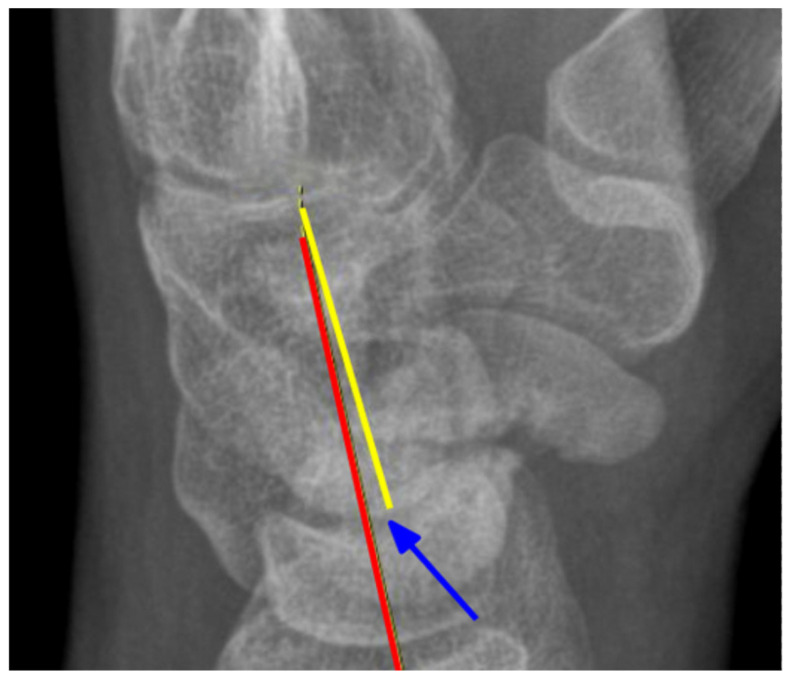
Measurement of the CLA angle. Blue arrow—angle, yellow line—the axis of the capitate, red line—the axis of the lunate. X-ray of the right hand, PA view.
